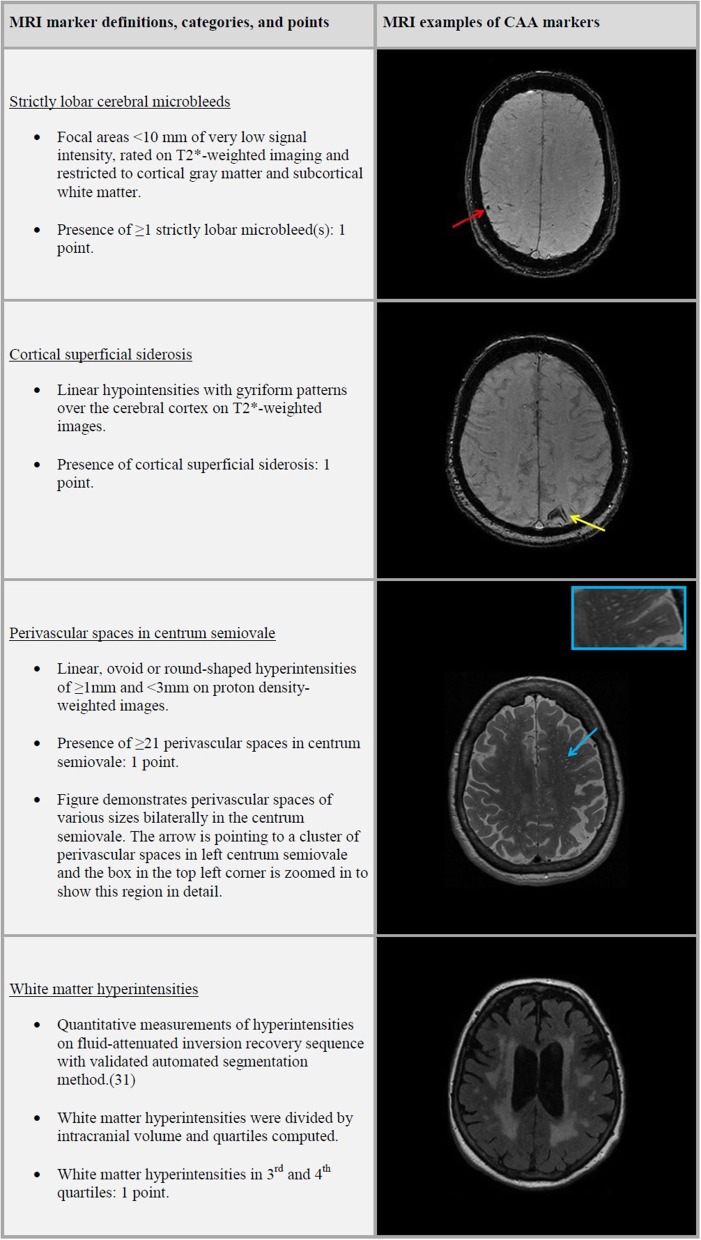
Magnetic Resonance Imaging (MRI) features of the cerebral amyloid angiopathy (CAA) score.
